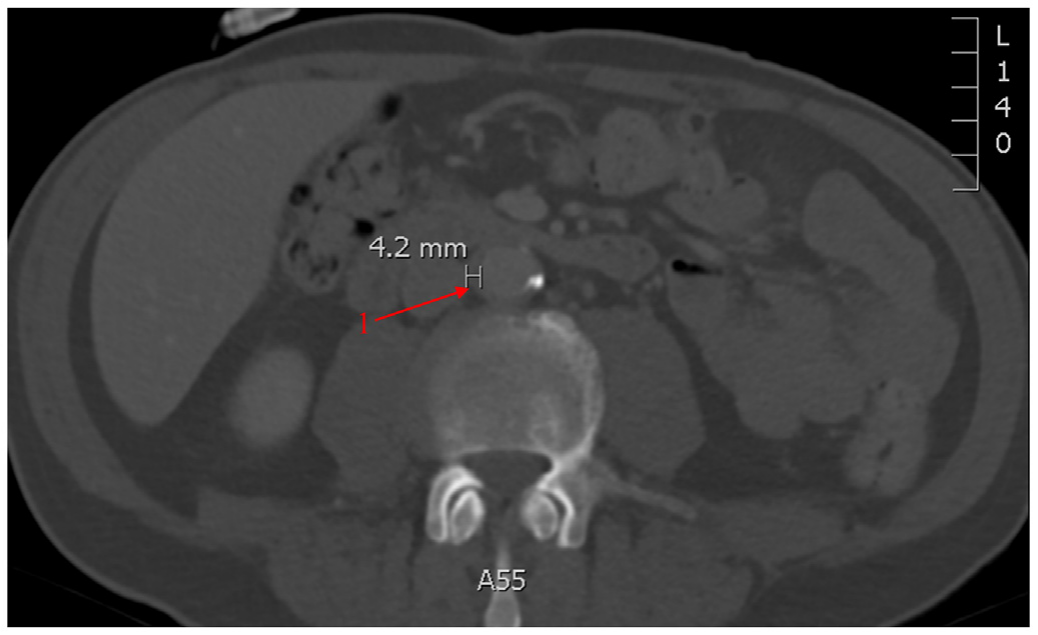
Enhanced computed tomography (CT) scan of the abdomen from May 2021. After 3 months of nivolumab therapy. Early infrarenal aortitis (1) with maximal aortic wall thickness measured at 4.2 mm. Mild aortic calcification is seen, which is stable from prior imaging.
